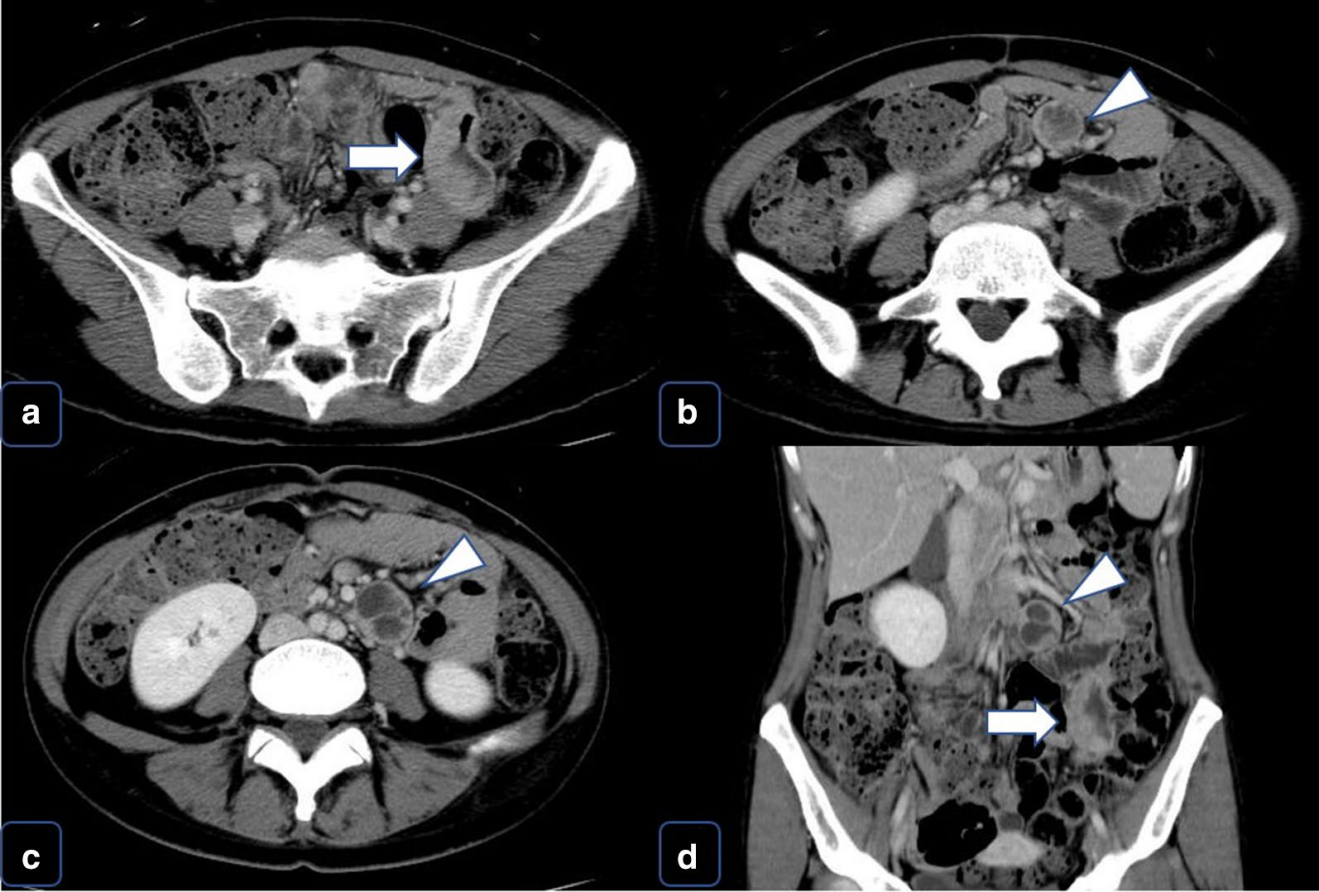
CT findings. a Horizontal view. Primary tumor (the white arrow). b, c Horizontal view. Lymph nodule (the arrowhead). d Sagittal view. Primary tumor (the white arrow) and lymph nodule (the arrowhead)
